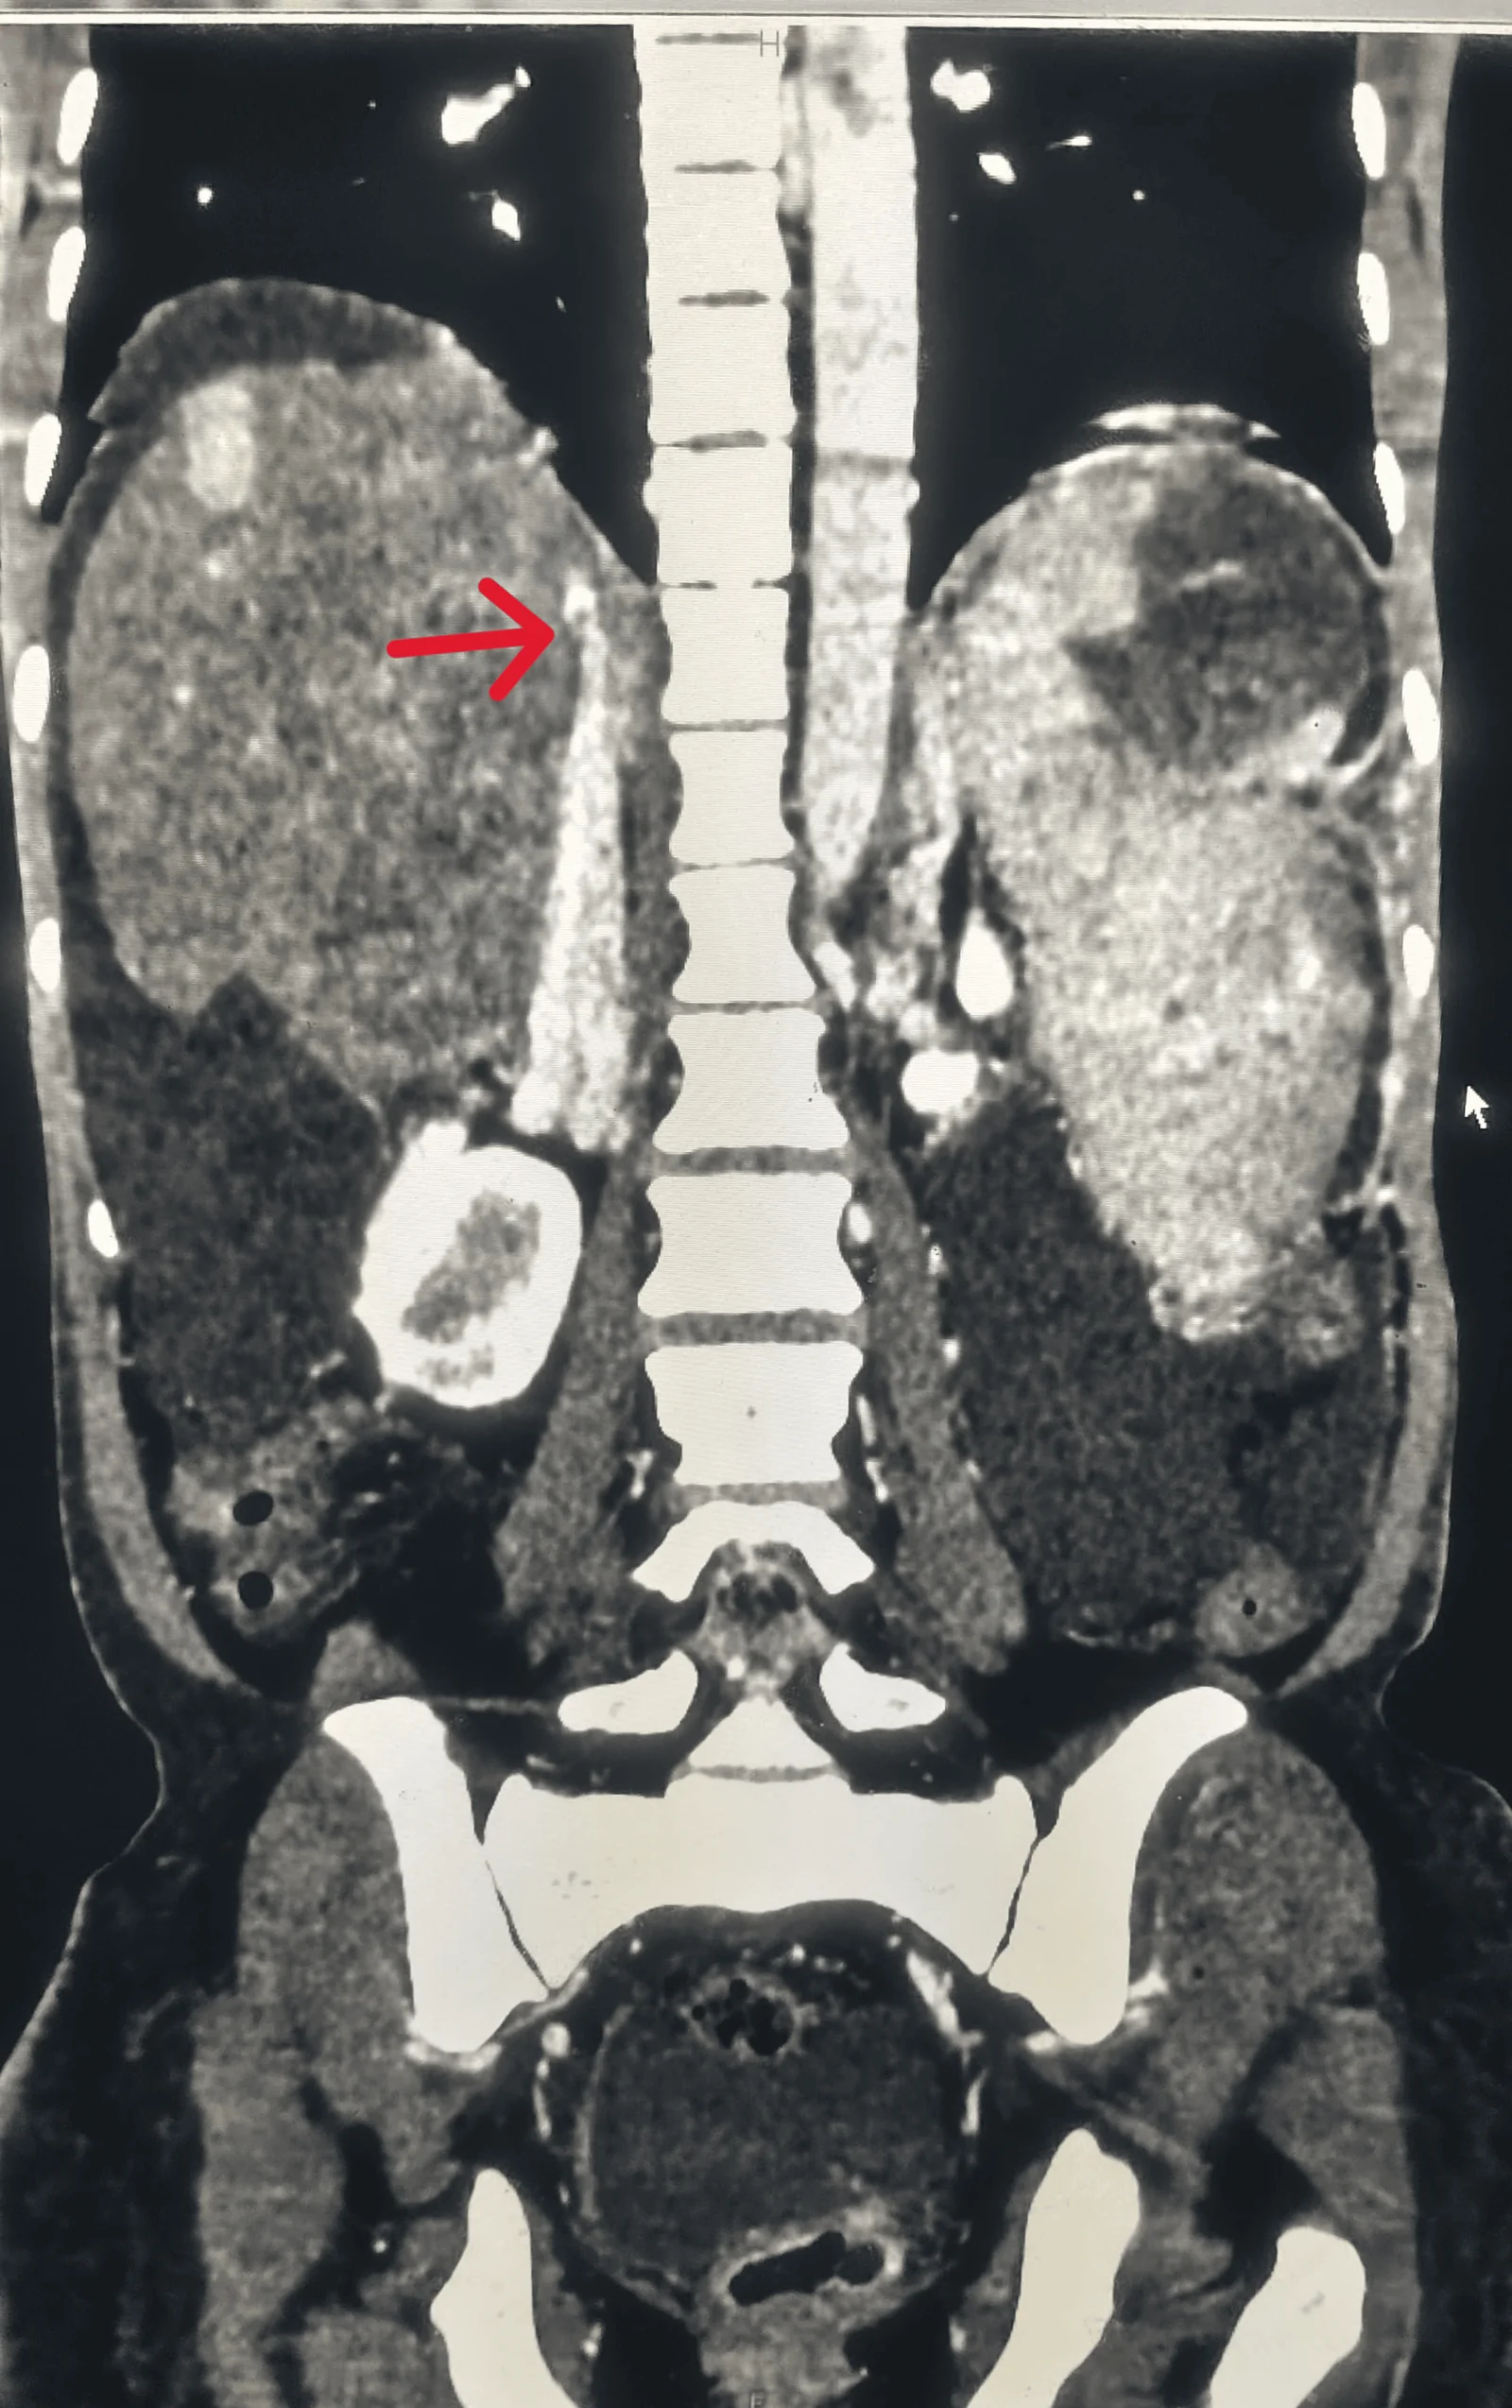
Coronal contrast-enhanced CT section of the upper abdomen demonstrating an attenuated intrahepatic inferior vena cava (red arrow) with non-visualisation of the hepatic veins, consistent with hepatic venous outflow obstruction.
